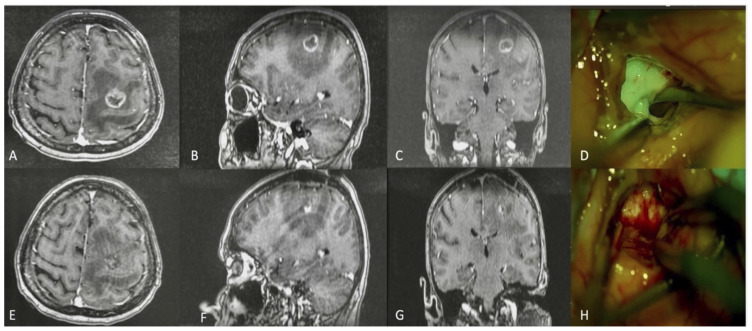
Intraoperative tumor visualization. Preoperative axial (A), sagittal (B), and coronal (C) gadolinium-enhanced MRI images demonstrating a tumoral lesion in the left premotor area in a 42-year-old male; (D): intraoperative real-time visualization of the tumor under the YELLOW 560-nm filter; (E–G): postoperative MRI confirmed total tumor resection; (H): cavity after tumor resection.
